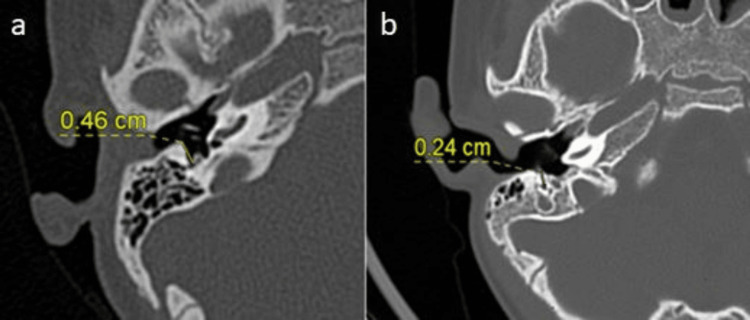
(a,b) Facial recess types HRCT of the temporal bone showing (a) wide facial recess (>3 mm) and (b) narrow facial recess (<3 mm). HRCT: high-resolution CT
